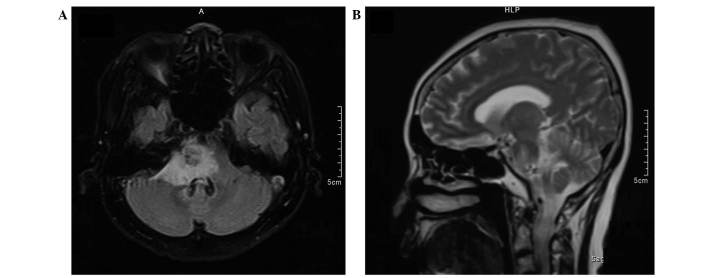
Magnetic resonance images obtained five months following stereotactic aspiration. The tumor size was 12×13×14 mm (volume, 1.2 cm^3^). New metastases are visible in the cerebellum. (A) Axial T1-weighted, fluid-attenuated inversion recovery image and (B) sagittal T2-weighted image.
